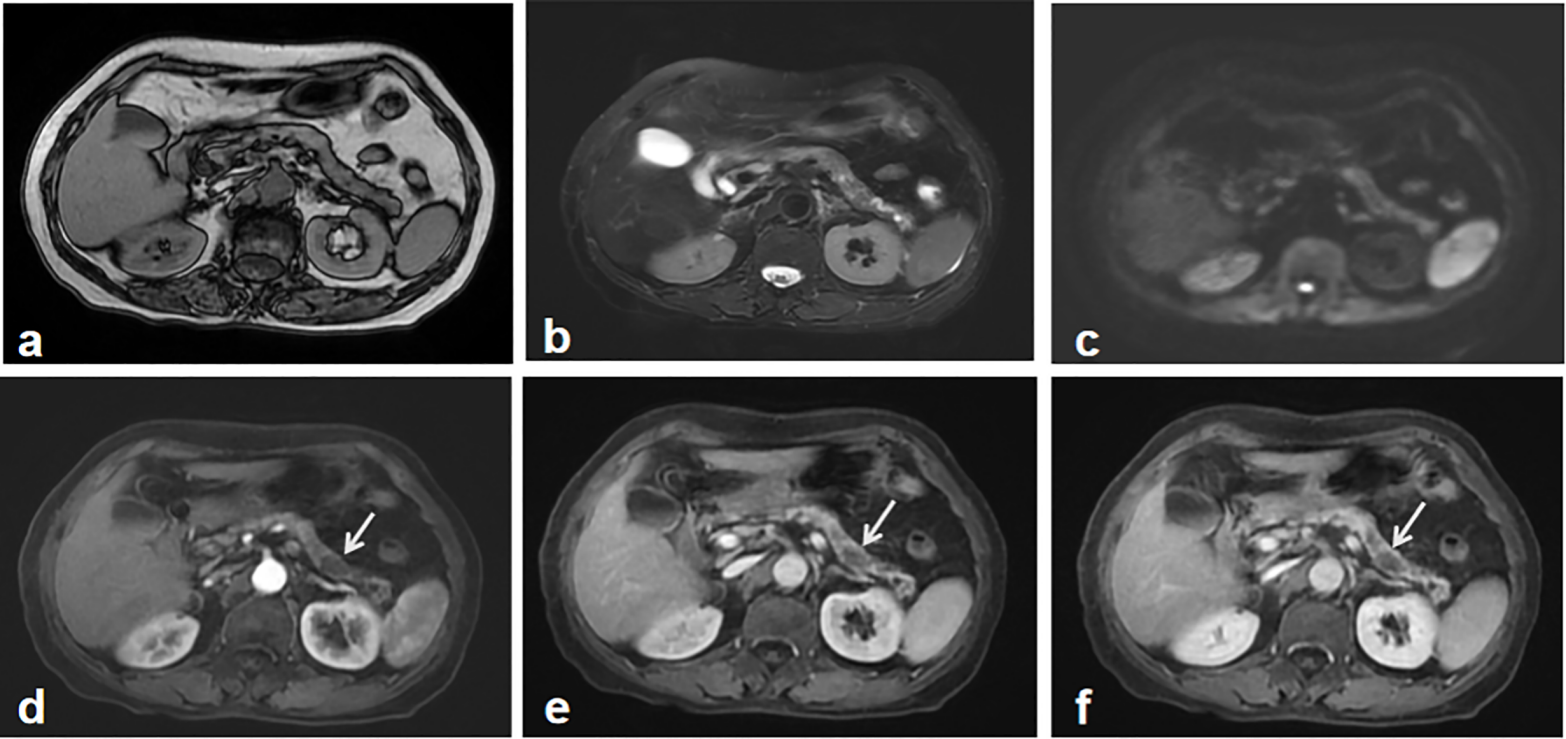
The first abdominal MRI. Both MR T1 (A) and T2 weighted image (B) showed the atrophy of the pancreas and inhomogeneous signal change in the body and tail of the pancreas. Diffusion weighted image didn’t show the apparent tumor mass-related signal change (C). Gadolinium-enhanced T1 weighted images including early arterial phase (D), late arterial phase (E) and portal phase images (F), depicted multiple irregular hypointense signals without demonstrating a typical tumor imaging characteristics, which was regarded as an indeterminate lesion in the pancreas. A recommendation of imaging follow-up was made for the patient.
